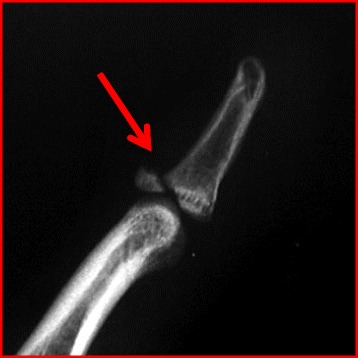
Lateral radiograph of a mallet finger with a bony avulsion fragment (*red arrow*)
